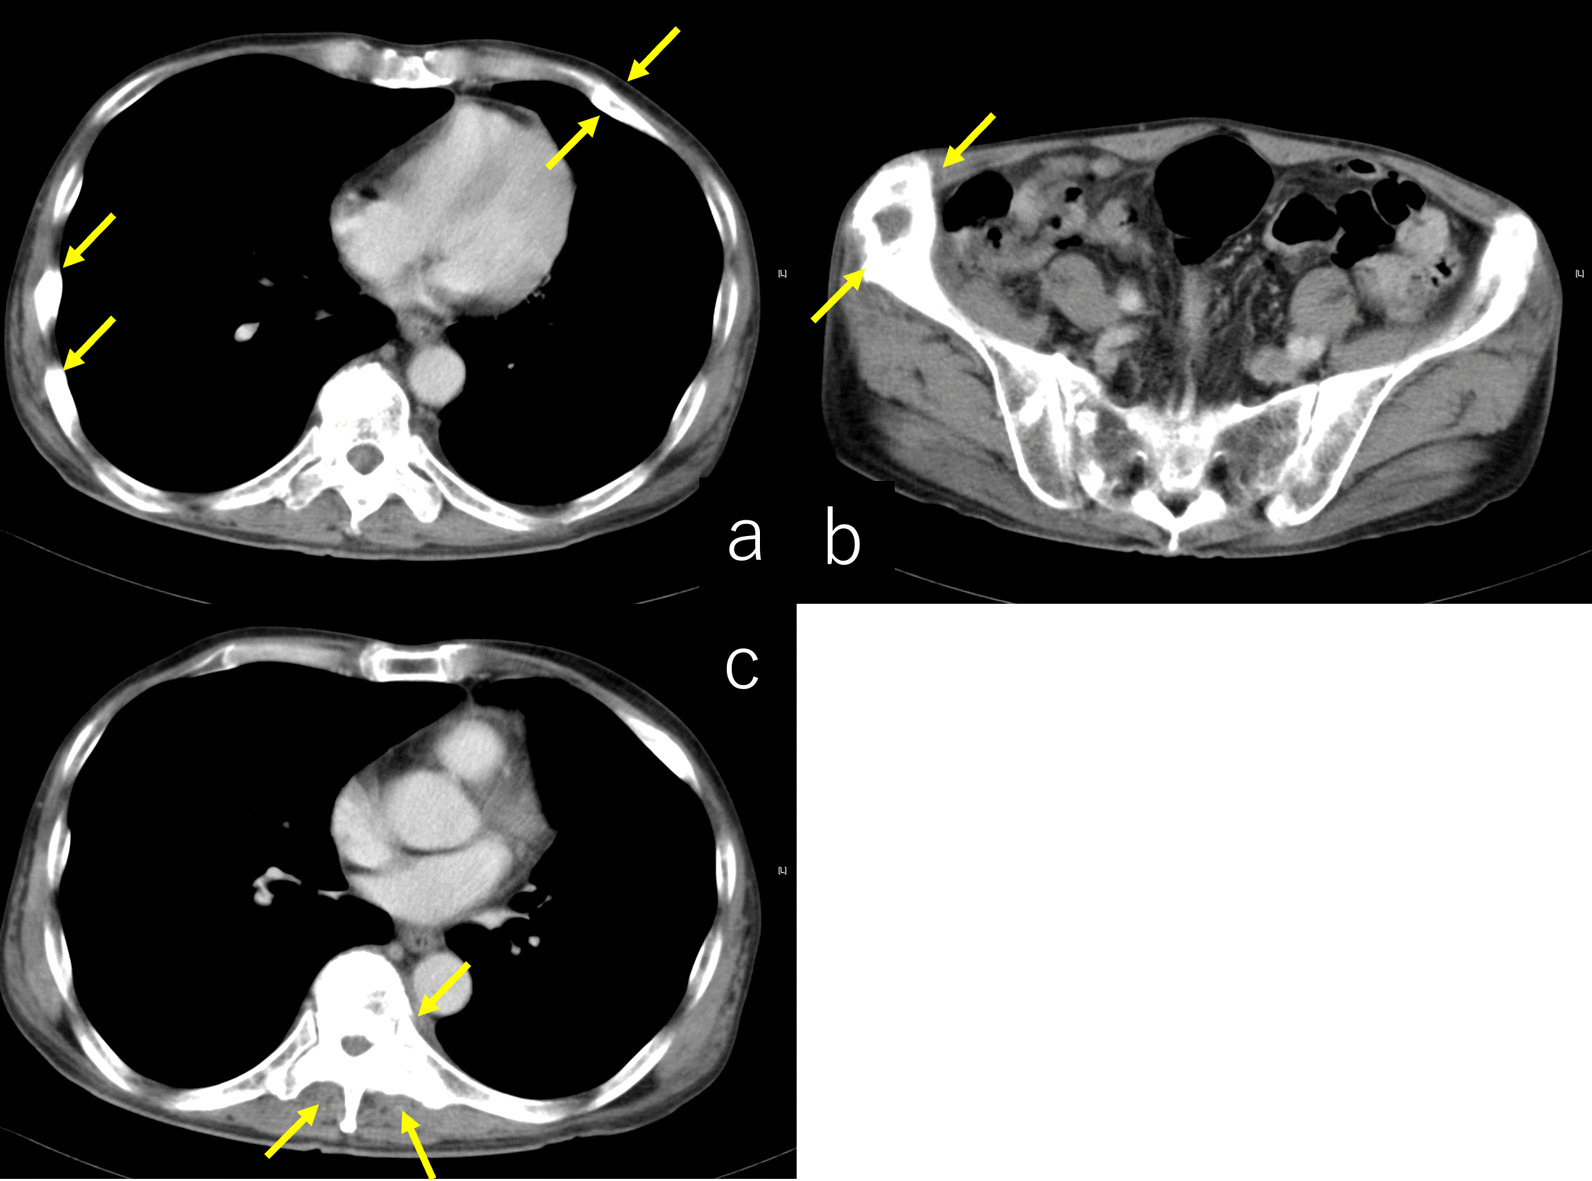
Computed tomography showed shrinkage of the lesion in the left eighth rib (a), right iliac bone (b), and ninth thoracic vertebra (c) (yellow arrows)
